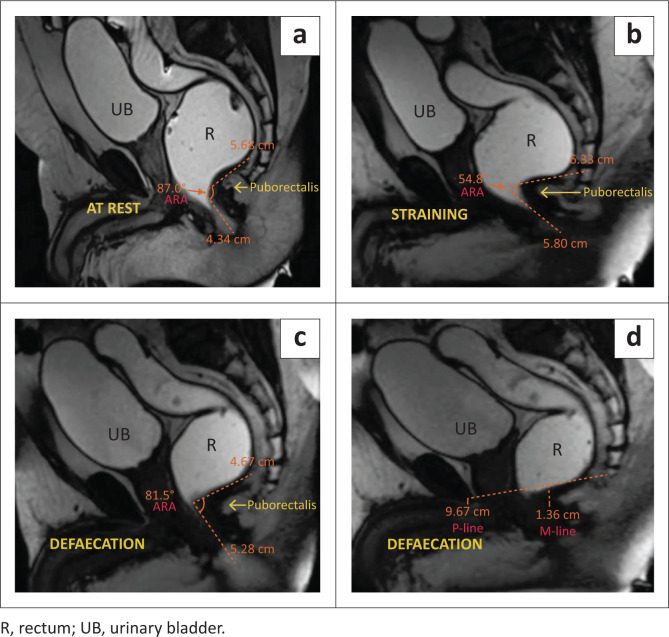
(a–d) MRI defaecogram: Spastic pelvic floor syndrome. (a) At rest shows the anorectal angle (ARA) at 87 degrees. (b) The straining phase shows a significant decrease in ARA to 54.8 degrees, with the puborectalis muscle indenting the anorectal junction. (c) Defaecation phase with an ARA of 81.5 degrees (less than that at rest), with persistent puborectalis indentation at the anorectal junction. (d) Defaecation phase showing Grade 1 anorectal descent.
